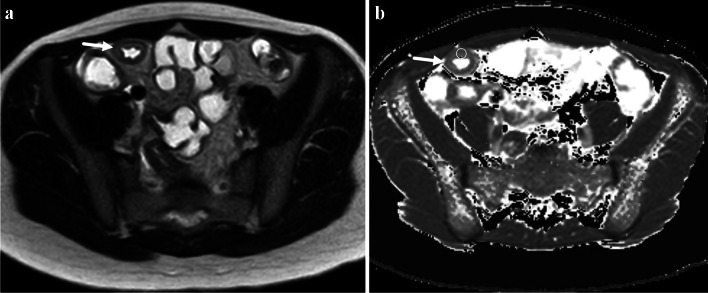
A 12-year-old female with Crohn’s disease. a Axial T2-weighted single-shot fast spin-echo MR image shows ileal wall thickening, intramural edema, and radiologic ulcers (*arrow*). b Axial T1 relaxation parametric map shows a region of interest (white circle) within the wall of the inflamed bowel (T1 = 1,361 ms) (*arrow*). Median bowel wall T1 relaxation time (ms) has been reported to be 1,159 (interquartile range 953 to 1,253) in healthy controls at 1.5-T [22]
